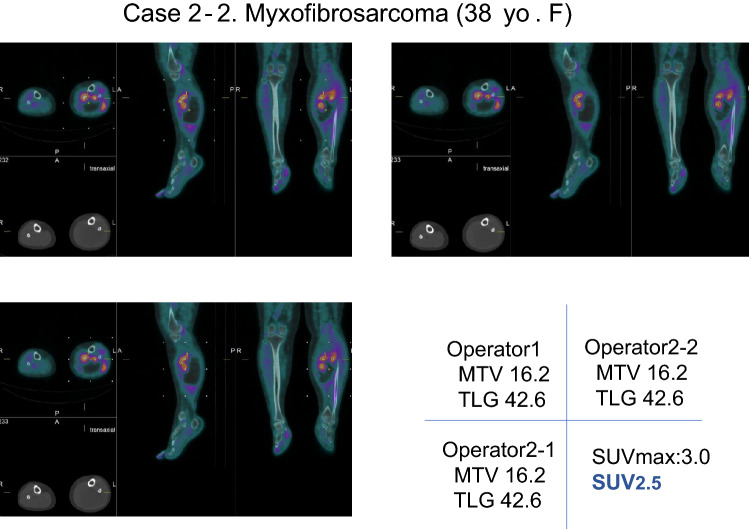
When the lower limit by the fixed value SUV 2.5 was used, the inter- and intra-operators MTV and TLG measurements were accorded
